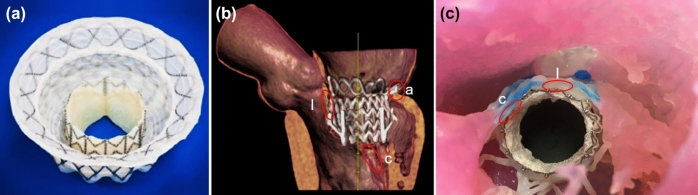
Bidirectional deformation of cardiac tissue and device. (a) Nitinol transcatheter mitral valve replacement (TMVR) device (Intrepid, Medtronic, USA); (b) Computed tomography image of the TMVR device implanted into a 3D printed mitral valve model incorporating tissue-specific material properties. (c) View from the left ventricle of the TMVR device deployed within a 3D printed mitral valve model. Regions of device and tissue deformation of the annulus (a), leaflet (l), and chordae tendineae (c) are identified (red ovals).
